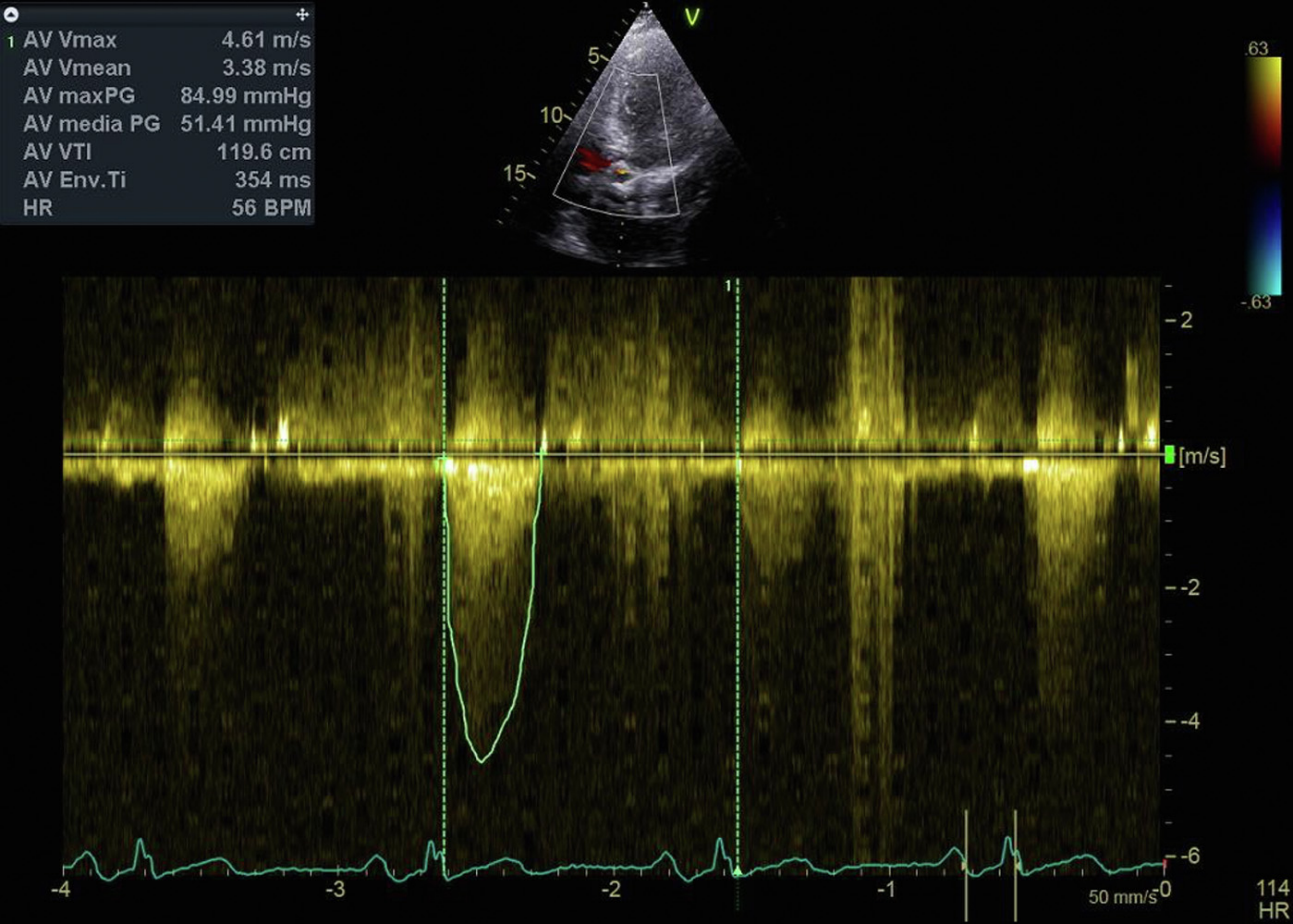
Continuous Doppler on Aortic Bioprosthesis Continuous Doppler shows a mean gradient of 51.41 mm Hg. AV = atrioventricular; BPM = beats/min; HR = heart rate; VTI = velocity time integral.
